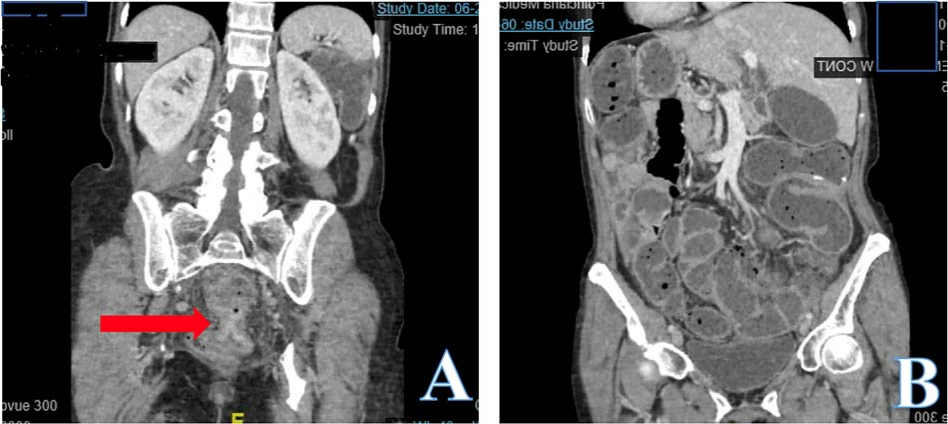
Abdomen and pelvis CT scan, coronal view (A) demonstrates low rectal concentric and symmetric near obstructing stricture indicated with the arrow. Coronal view (B) demonstrates a diffuse colonic and small bowel dilation consistent with LBO.
